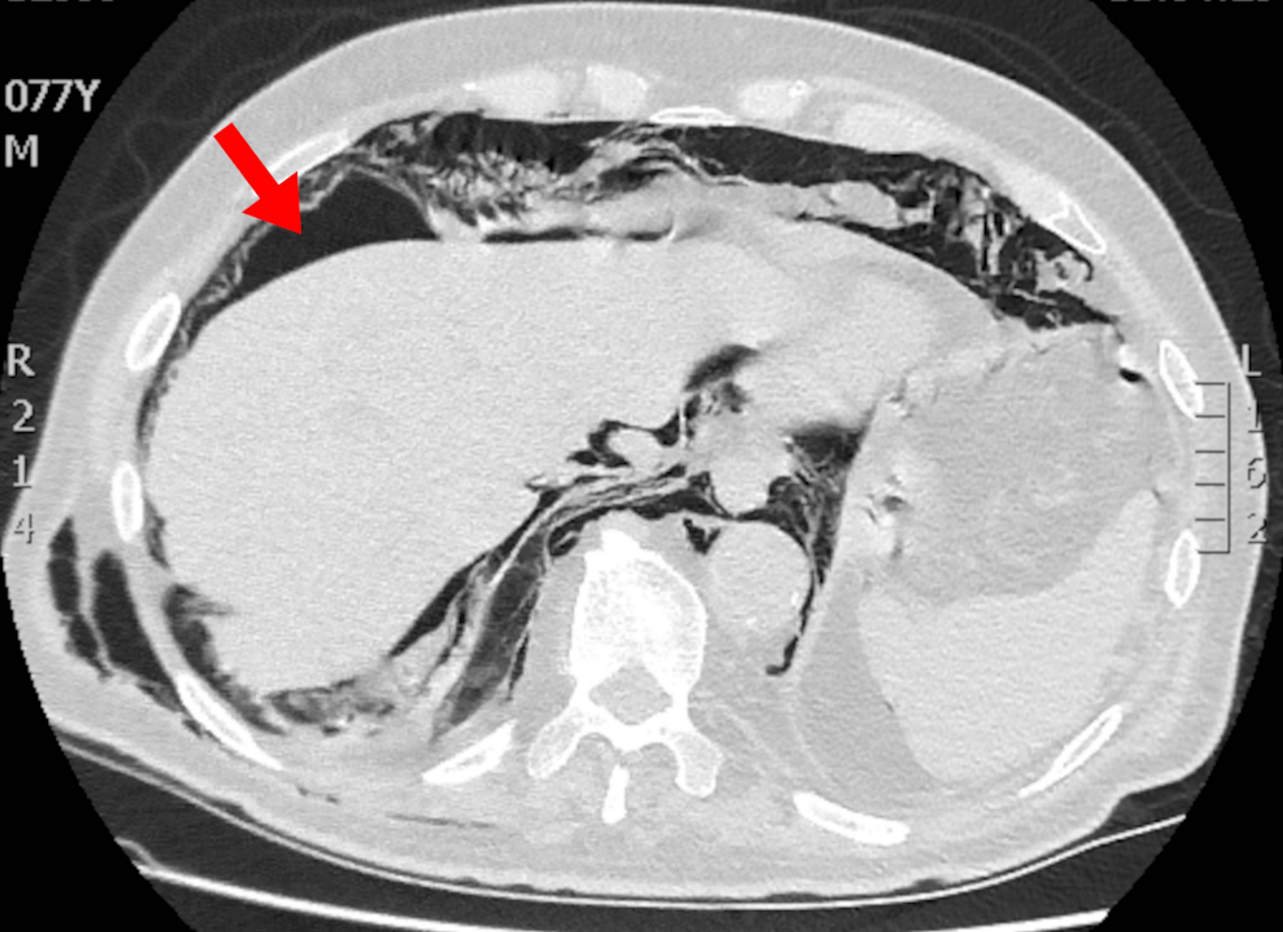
CT of the abdomen showing significant free intraperitoneal air (red arrow points to the pneumoperitoneum)
